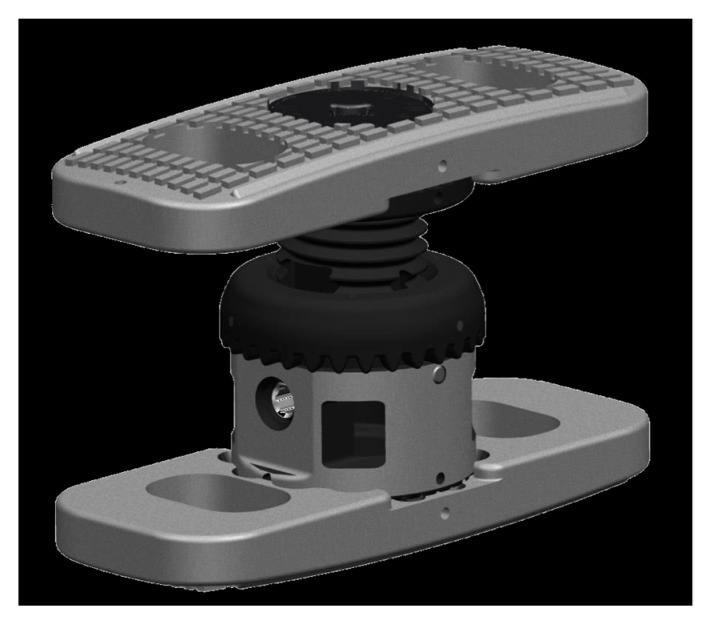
Prototype of the non-metallic, X-ray-translucent CF/PEEK expandable VBR. icotec, Altstätten, Switzerland.
